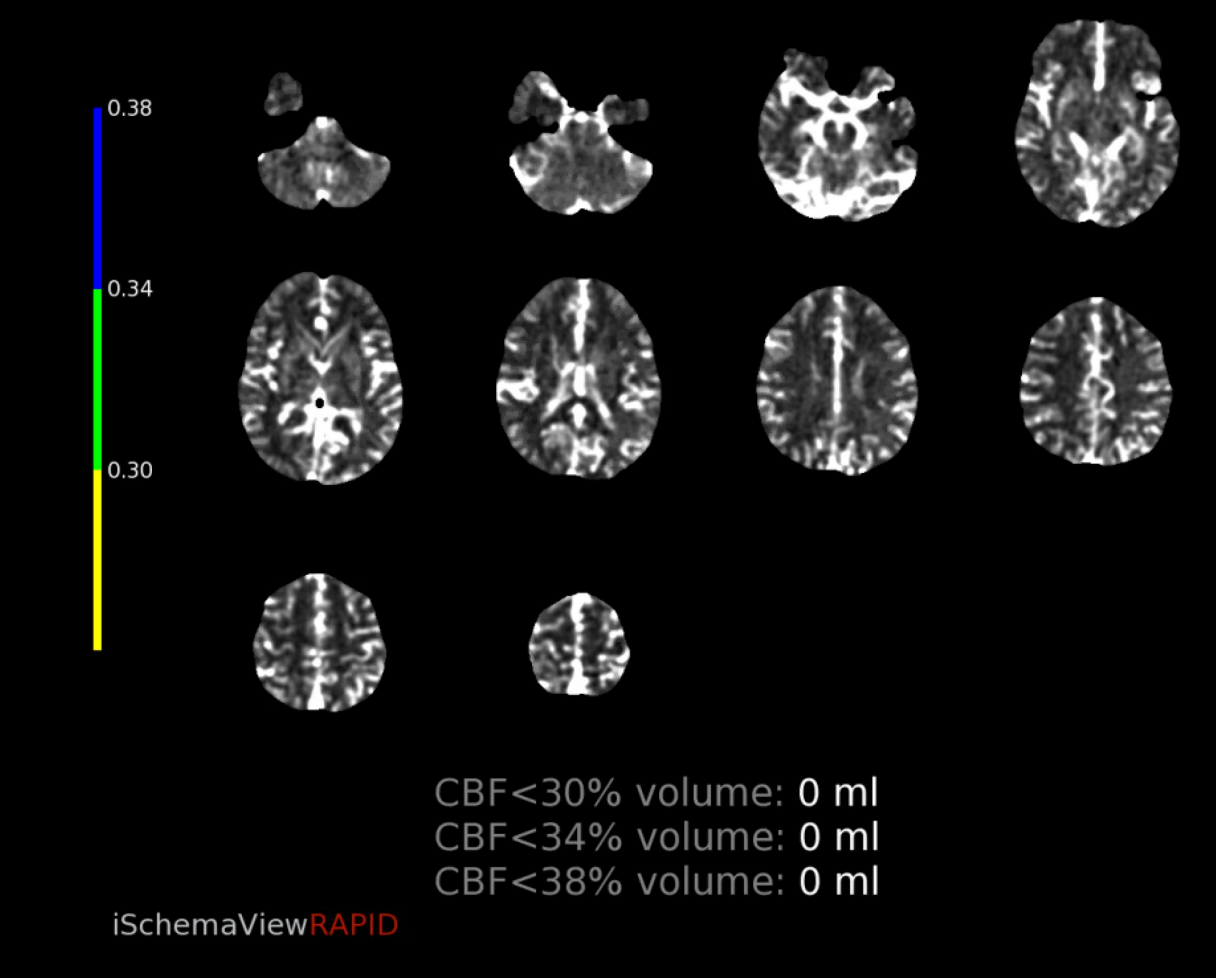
CT perfusion demonstrating normal CBF CBF=Cerebral blood flow
